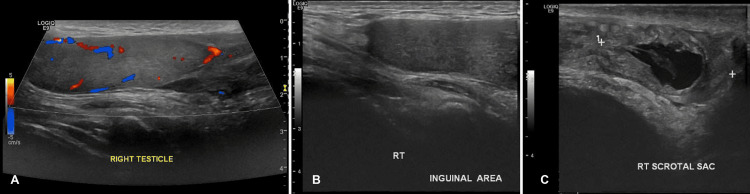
Preoperative Doppler ultrasound - case 1 The images show the right testis (panel A) in the inguinal canal (panel B) with good vascularity. Panel C depicts the scrotal sac
